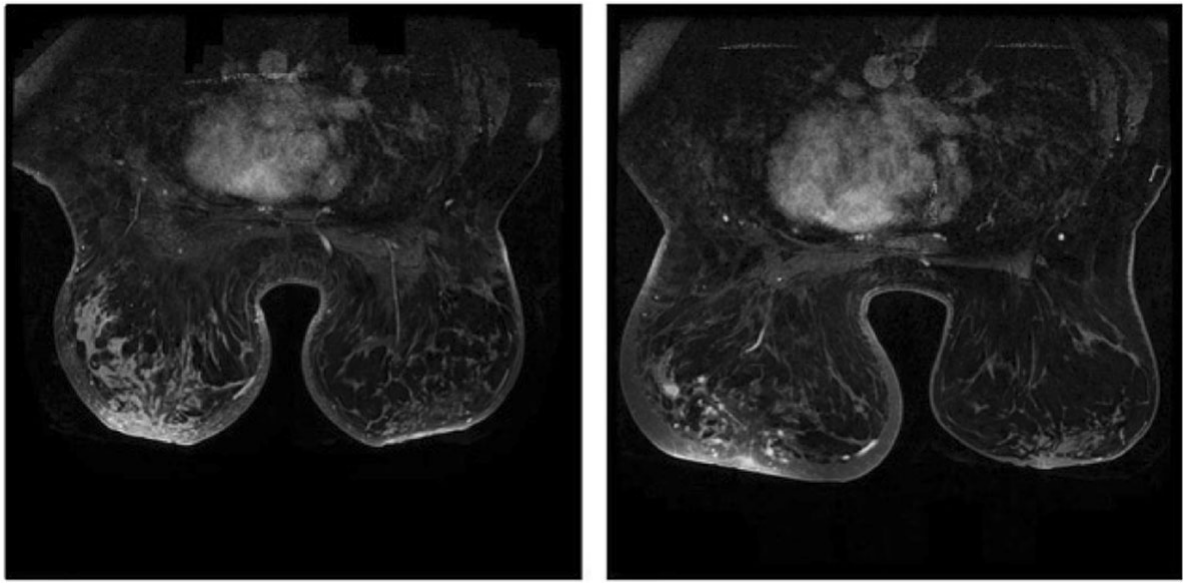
Diffused tumors enhanced in breast MRI performed before (left image) and after (right image) neoadjuvant chemotherapy
